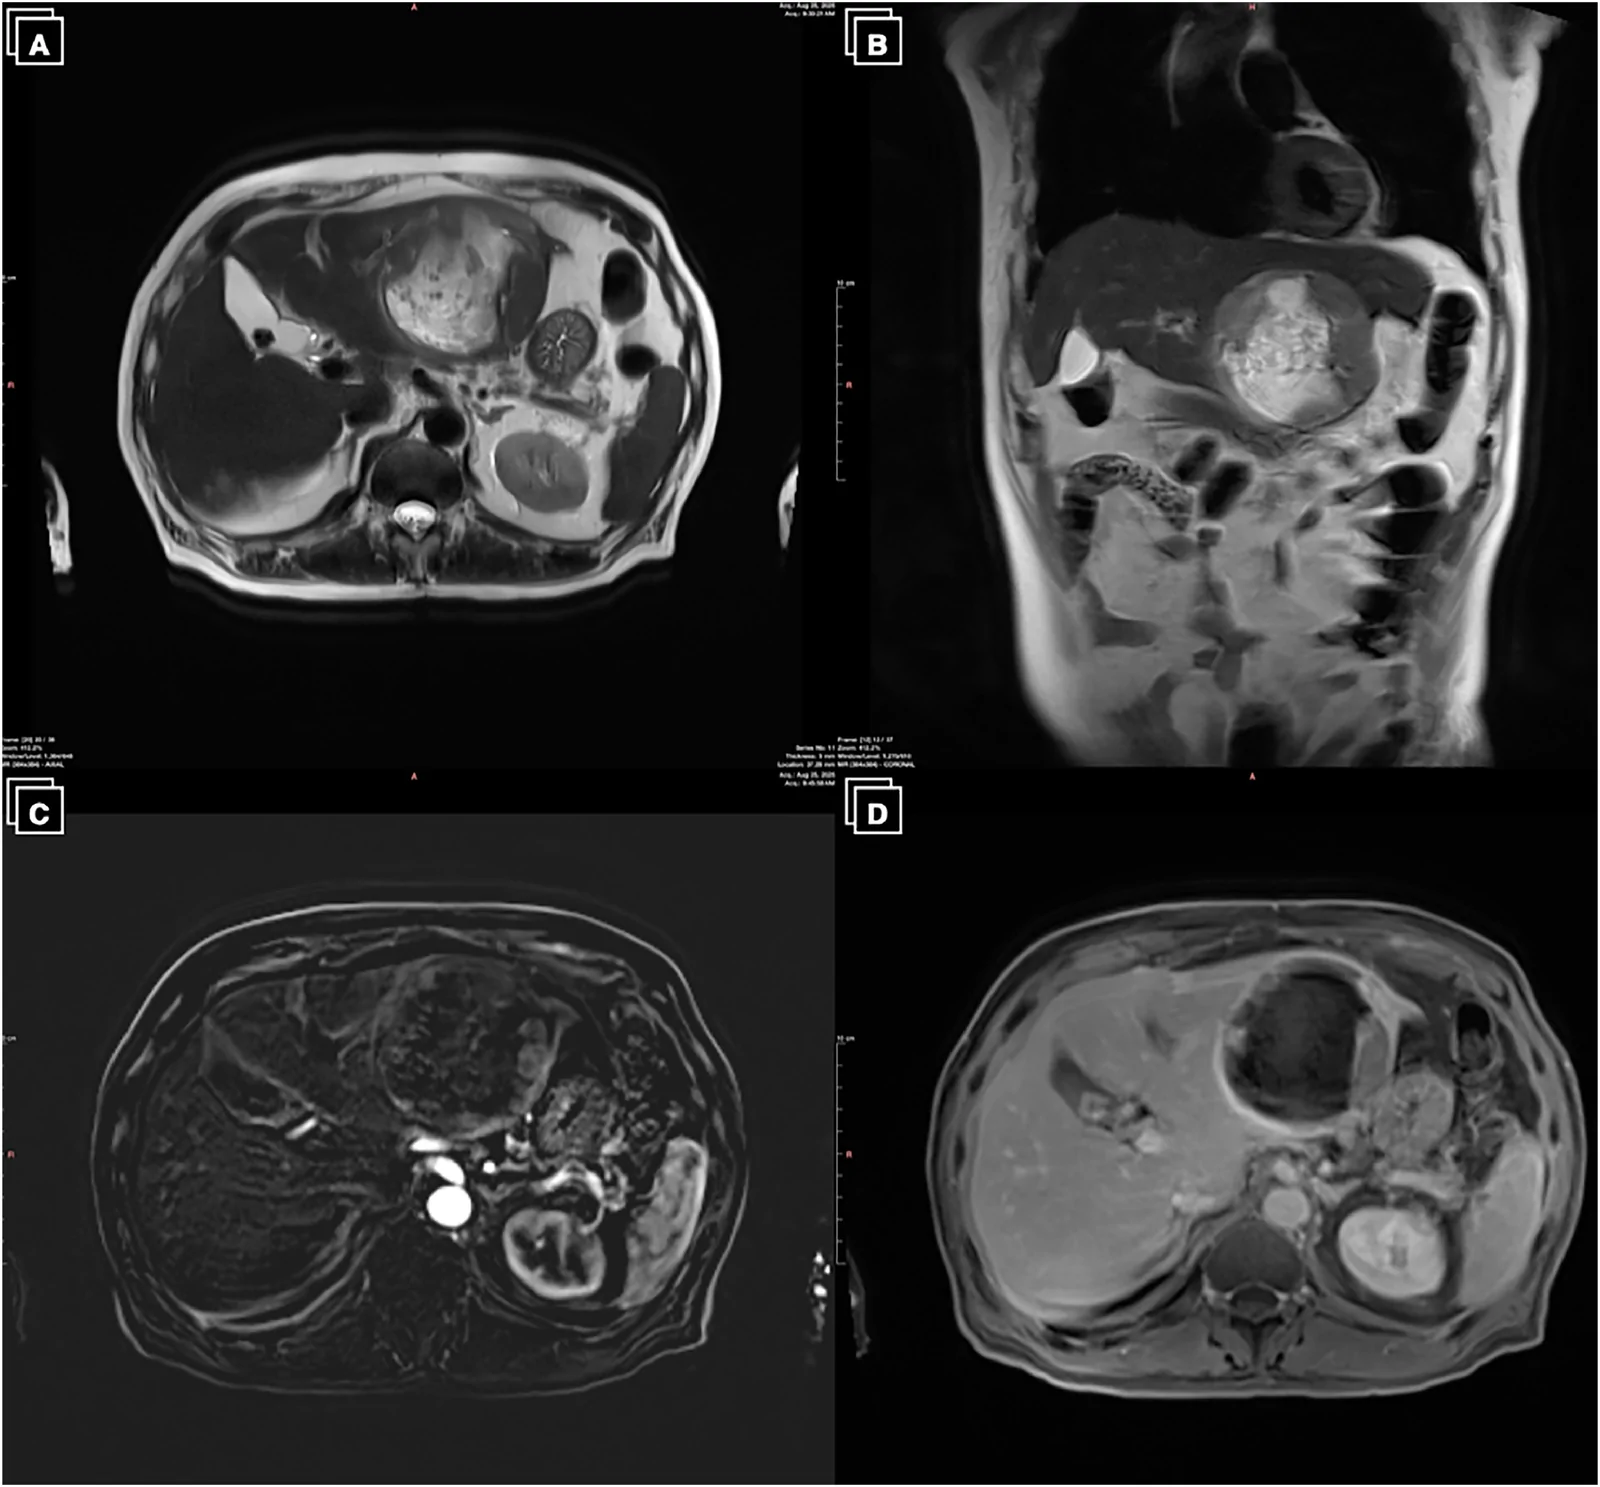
Follow-up MRI approximately 6 weeks after embolization. (A) Axial T2-weighted HASTE MRI obtained August 25 demonstrates a heterogeneous post-treatment cavity in the left hepatic lobe containing mixed signal material, compatible with necrotic debris and hemorrhagic or proteinaceous contents. (B) Coronal T2-weighted HASTE MRI further demonstrates the treated cavity and peripheral rim-like signal abnormality. (C) Axial early postcontrast subtraction image demonstrates no convincing central enhancement within the treated cavity, with peripheral rim-like enhancement along the treatment margin. (D) Delayed postcontrast MRI demonstrates persistent peripheral rim-like enhancement without convincing central enhancement.
